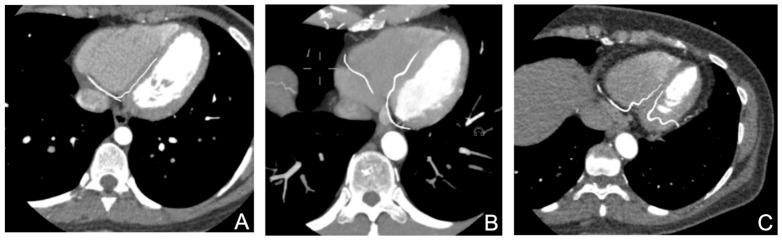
Coronary dominance on CCTA. (A) Right coronary dominance. (B) Left coronary dominance. (C) Codominance. Images were obtained utilizing maximum intensity projection (MIP) reconstructions on a 160-slice CT scanner at Clínica Reina Sofía.
